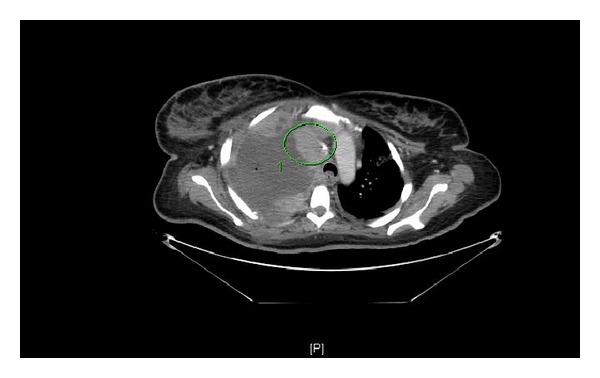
CT with contrast demonstrating near-complete superior vena cava occlusion by tumor invasion/extrinsic mass effect (21MAR2010).
